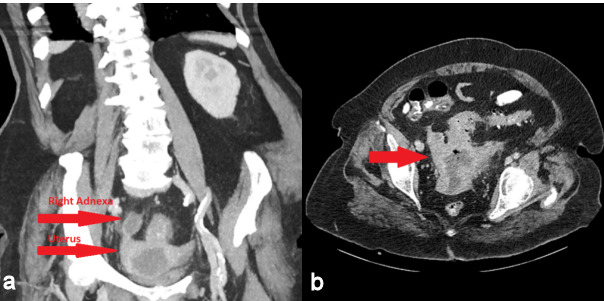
Computer tomography (CT) a) of the abdomen in the coronal plane with intravenous contrast, b) of the lower pelvis in the axial plane with intravenous contrast; air bubble in the uterine cavity and right adnexa
